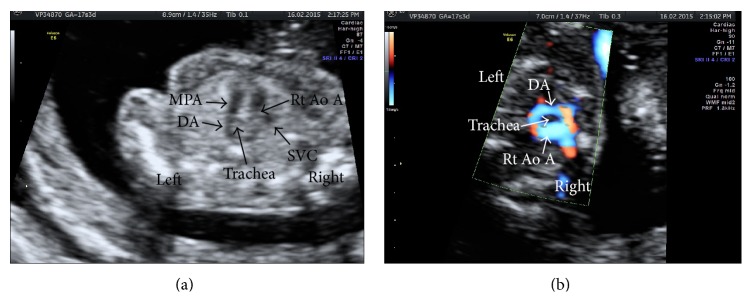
Fetal echocardiography at 17 weeks' gestation. Two-dimensional gray-scale (a) and high definition color Doppler (b) images in three-vessel and trachea view. The right aortic arch (Rt Ao A) and ductus arteriosus (DA), forming classic “U” shape. MPA, main pulmonary artery; SVC, superior vena cava.
